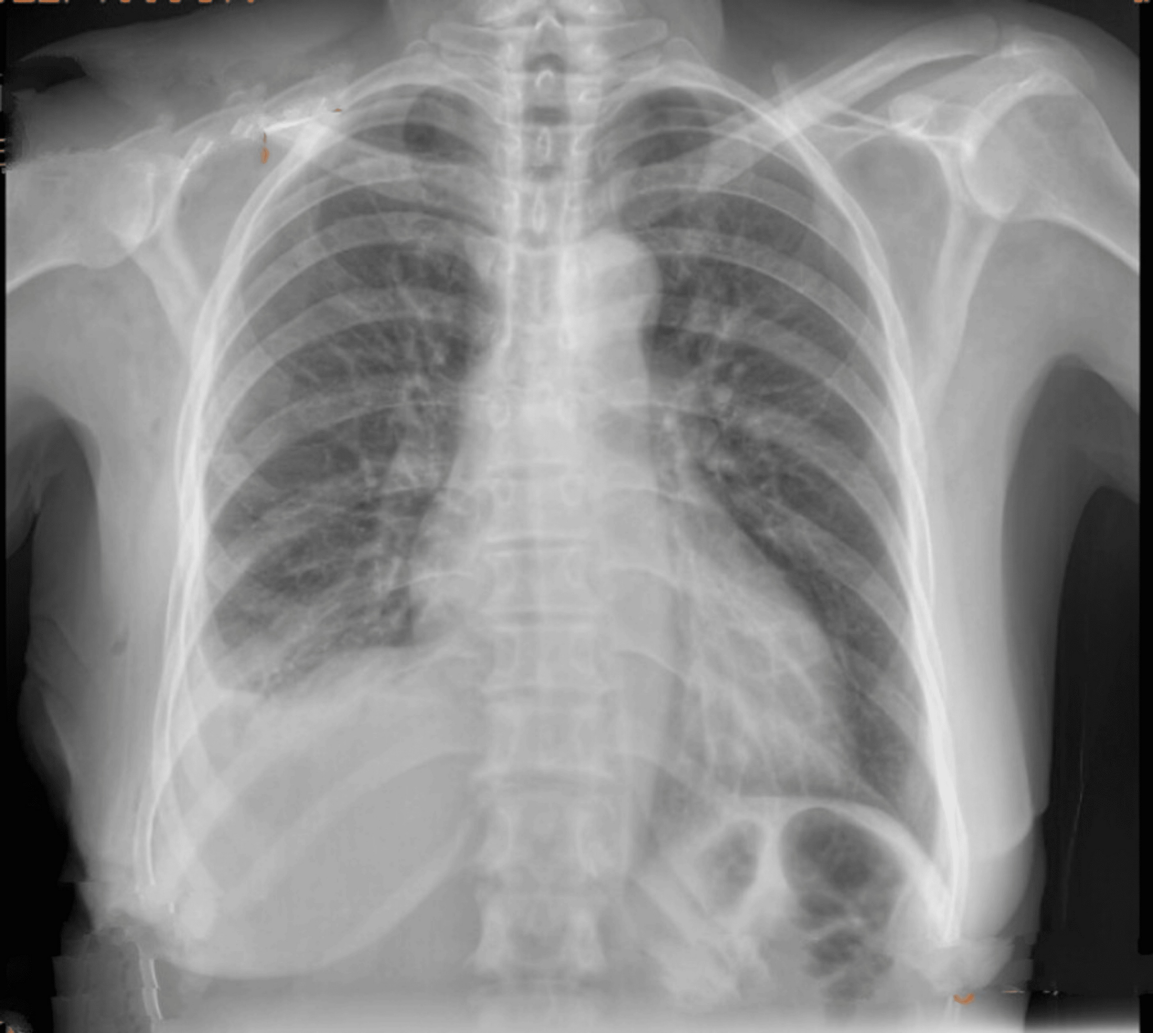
Chest X-ray at discharge showing complete resolution of the hydrothorax.
